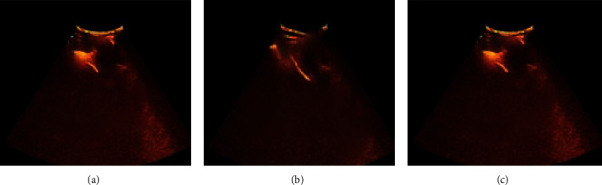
Contrast-enhanced ultrasound of drainage tube after puncture: (a–c) the contrast-enhanced ultrasound images of three patients after the PTGD.
